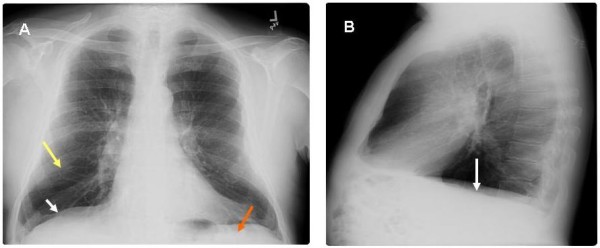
With A showing PA and B showing lateral views of the chest roentgenograms of the same patient. The arrow marks refer to the flattening of the diaphragm (white arrows), emphysematous changes (yellow arrow) and the decreased zone of apposition (red arrow).
